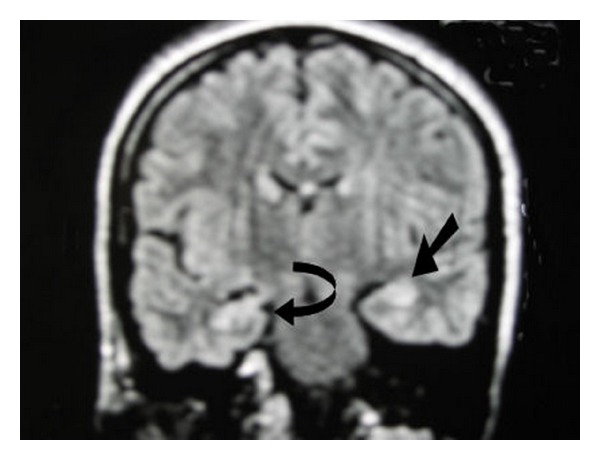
MRI showing findings suggestive of hypoglycemia.
